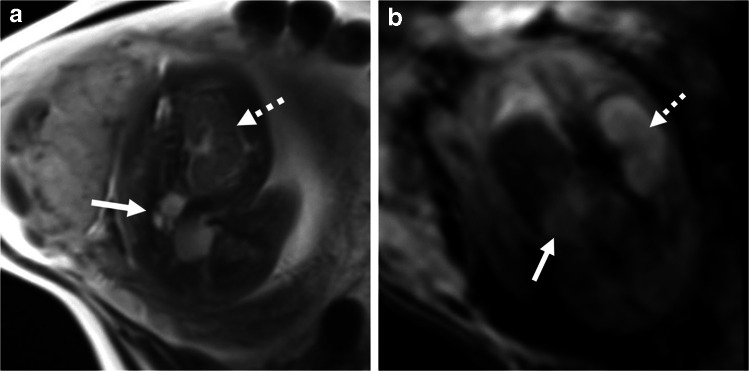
a Fetal MRI of a 29-week gestation male fetus. Oblique coronal Single-Shot Fast Spin Echo (SSFSE) images demonstrate an ectopic right multicystic dysplastic kidney (MCDK) (arrow) located in the pelvis and the orthotopic position of the left kidney (dotted arrow). b Oblique coronal diffusion-weighted imaging (DWI) showing restricted diffusion of the pelvic tissue (arrow) confirming this as renal tissue. The left kidney is also showing restricted diffusion (dotted arrow)
